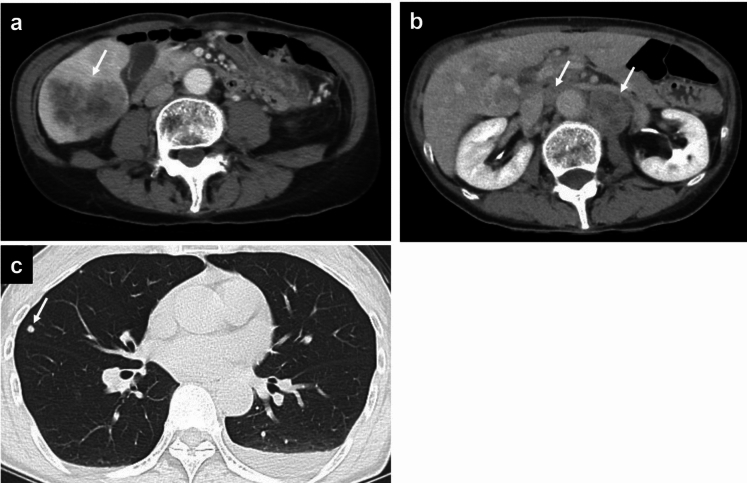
Abdominal and chest contrast-enhanced computed tomography (CT). a A 6-cm-diameter liver tumor in segment 6 is observed in the portal venous phase with contrast-enhanced CT (arrow). b A swollen para-aorta lymph node is observed in the equilibrium phase with contrast-enhanced CT (arrows). c Lung metastases are observed with chest CT (arrow)
